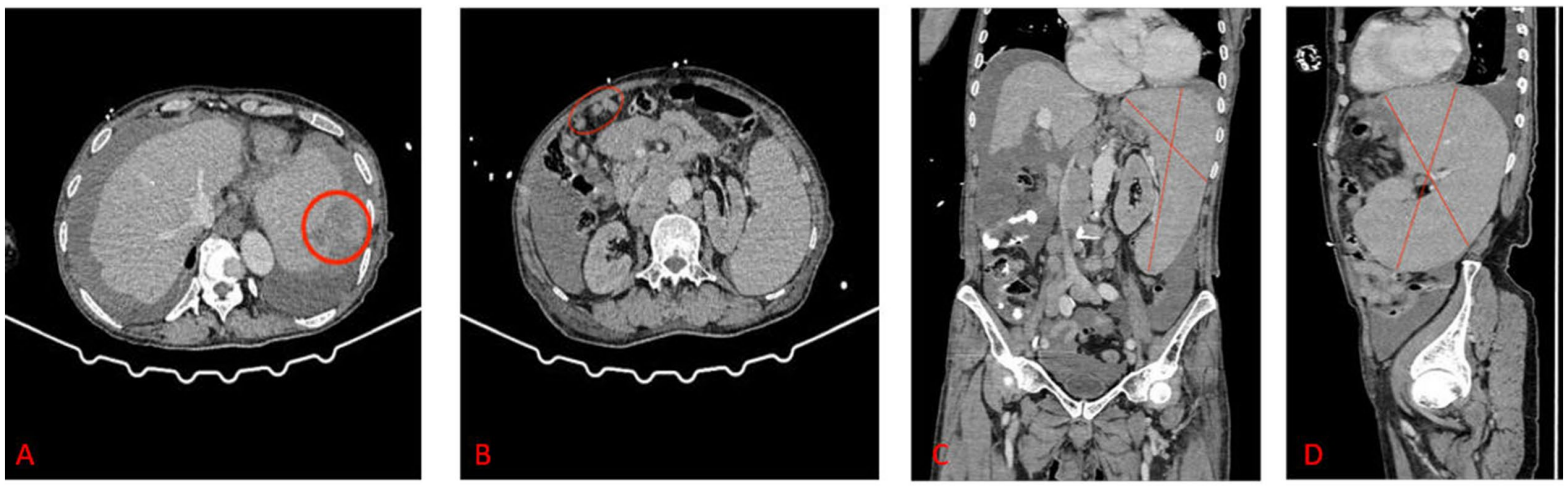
CT scan indicated a rupture at the superior pole of the spleen, hemoperitoneum, and multiple enlarged lymph nodes. (A) The red circle indicated the rupture point. (B) The red oval area indicated multiple enlarged lymph nodes. (C) Massive splenomegaly in the coronal plane (the red-line marked area). (D) Massive splenomegaly in the sagittal plane (the red-line marked area).
